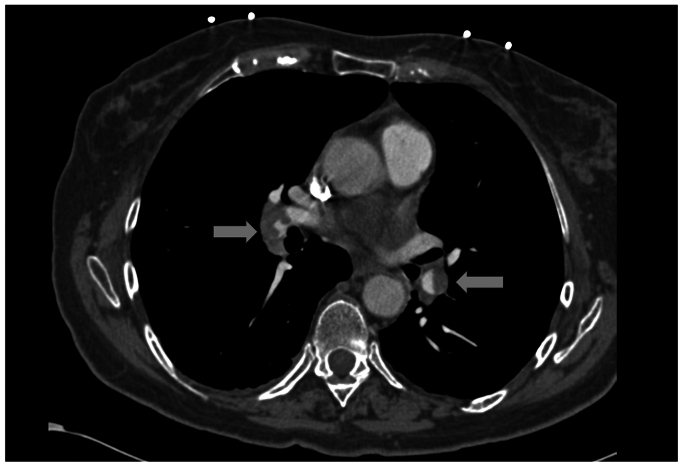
Computed tomography angiogram demonstrating bilateral pulmonary emboli.
